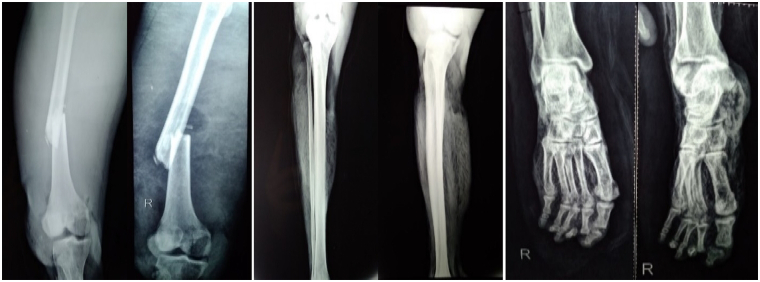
Anteroposterior (AP) and lateral (L) X-Ray view of the right lower extremity. Left: AP/L X-Ray view of the upper leg. Middle: AP/L X-Ray view of the lower leg. Right: AP/Oblique X-Ray view of the foot.
